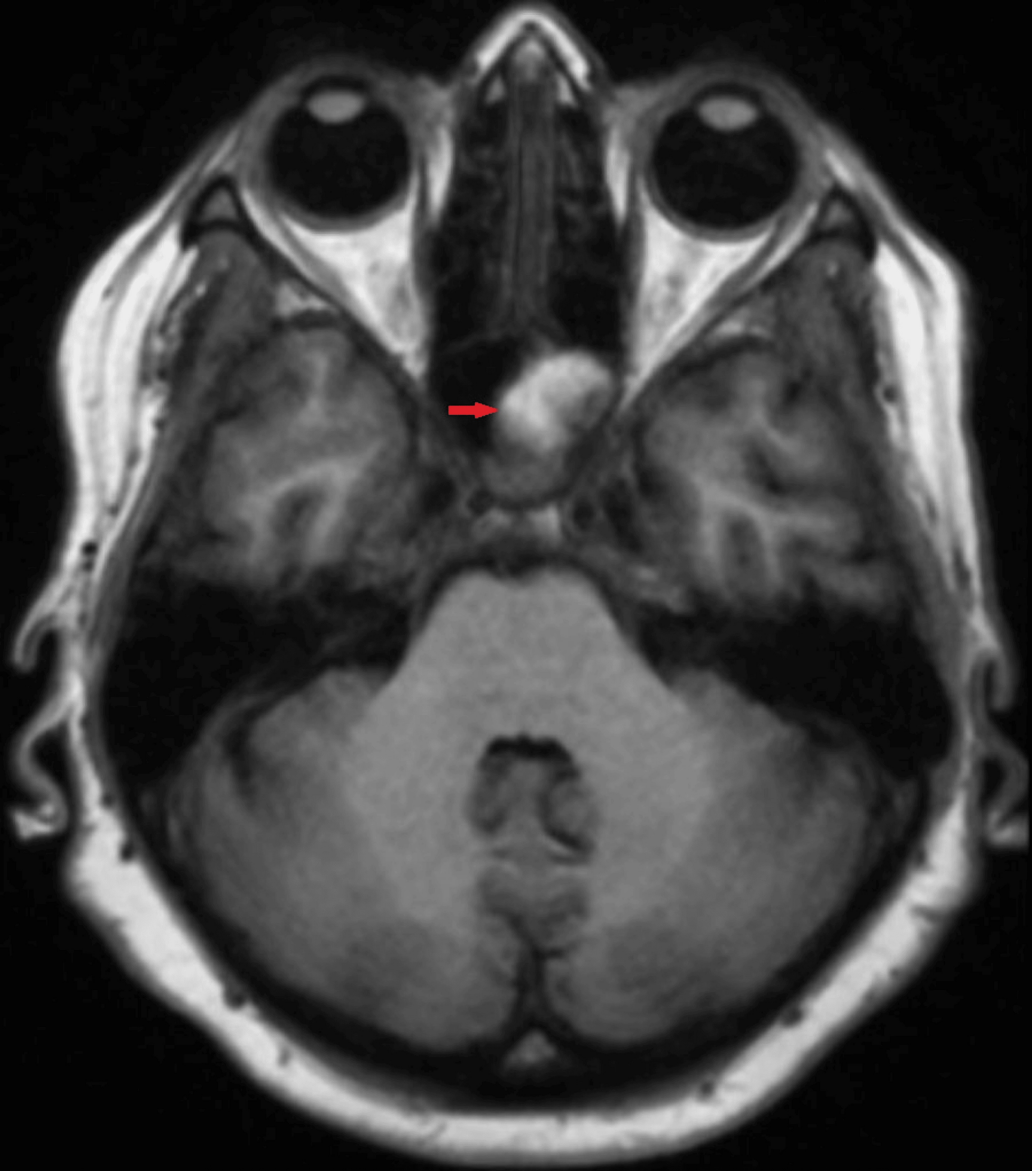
Magnetic resonance imaging, T2-weighted axial view. The red arrow indicates the sphenoid sinus, which demonstrates T1 hyperintensity along with a blooming artifact, without any expansion of the sinus
